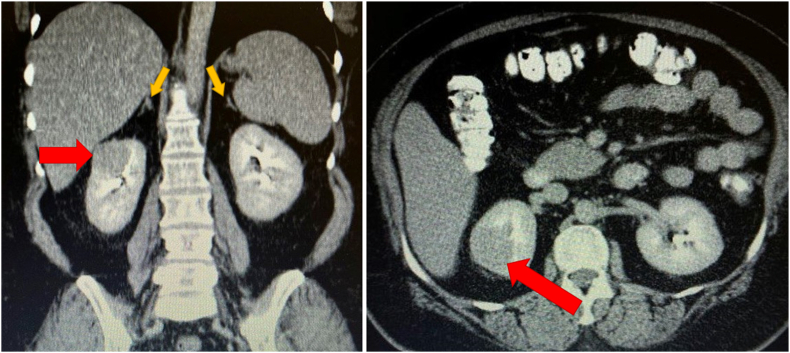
Abdominopelvic CT scan with intra-venous and oral contrast, showing right renal mass (red arrows) and normal adrenal glands (yellow arrows). (For interpretation of the references to colour in this figure legend, the reader is referred to the Web version of this article.)
